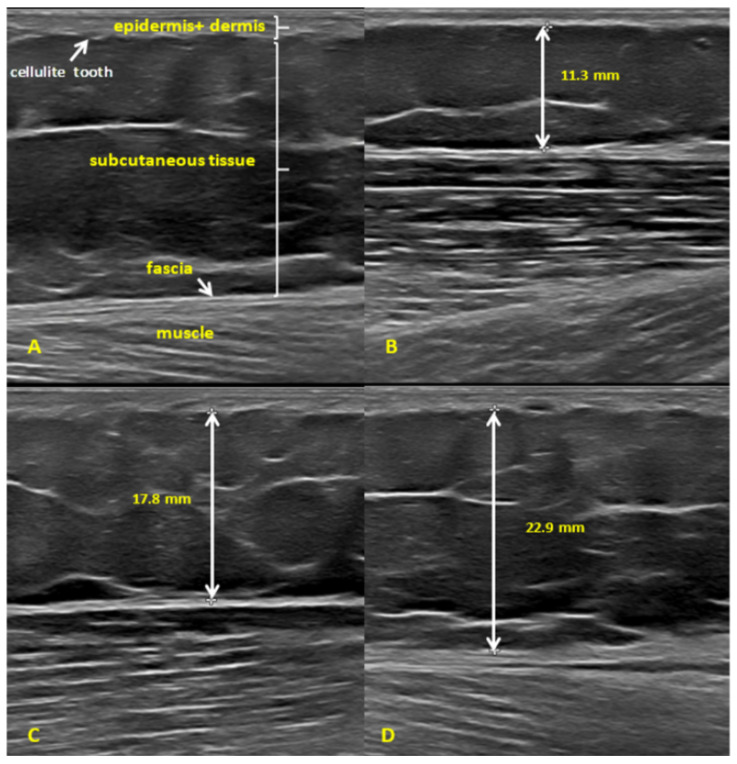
Ultrasound assessment of subcutaneous tissue (A). Subcutaneous tissue thickness: (B)—group 1; (C)—group 2; and (D)—group 3.
